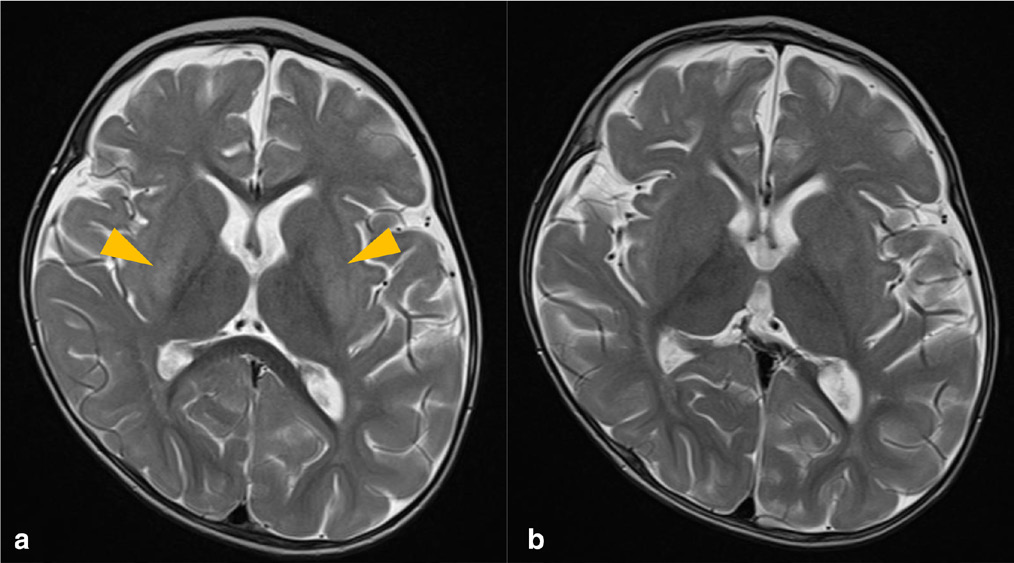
T*_2_-weighted axial MRI sequences demonstrating high signal within the putamina bilaterally (orange arrowheads) (A), which has subsequently resolved upon follow-up imaging two months later (B).
